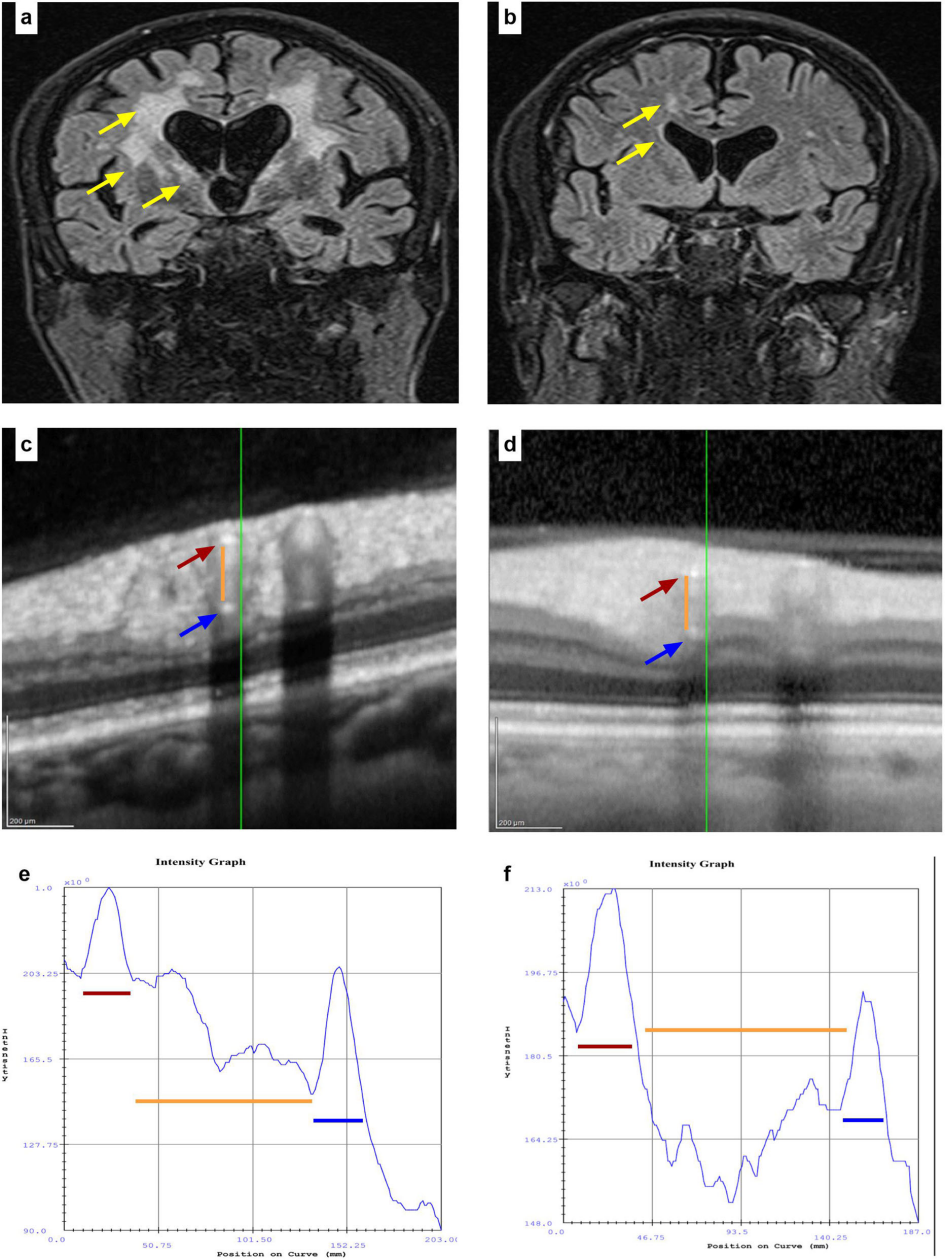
White matter hyperintensities (WMH) on FLAIR imaging and corresponding optical coherence tomography (OCT) scans and intensity graphs. a and b Examples of two patients with different extent of WMH on FLAIR imaging (yellow arrows), left Fazekas grade 3, right Fazekas grade 1, respectively. c and d OCT scans showing superior temporal branch of the retinal artery (red arrow: inner wall. Blue arrow: outer wall. Orange line: lumen). e and f intensity graphs showing the inner wall’s (red line) and the outer wall’s peak (blue line) with space in between (orange line) corresponding to the lumen
